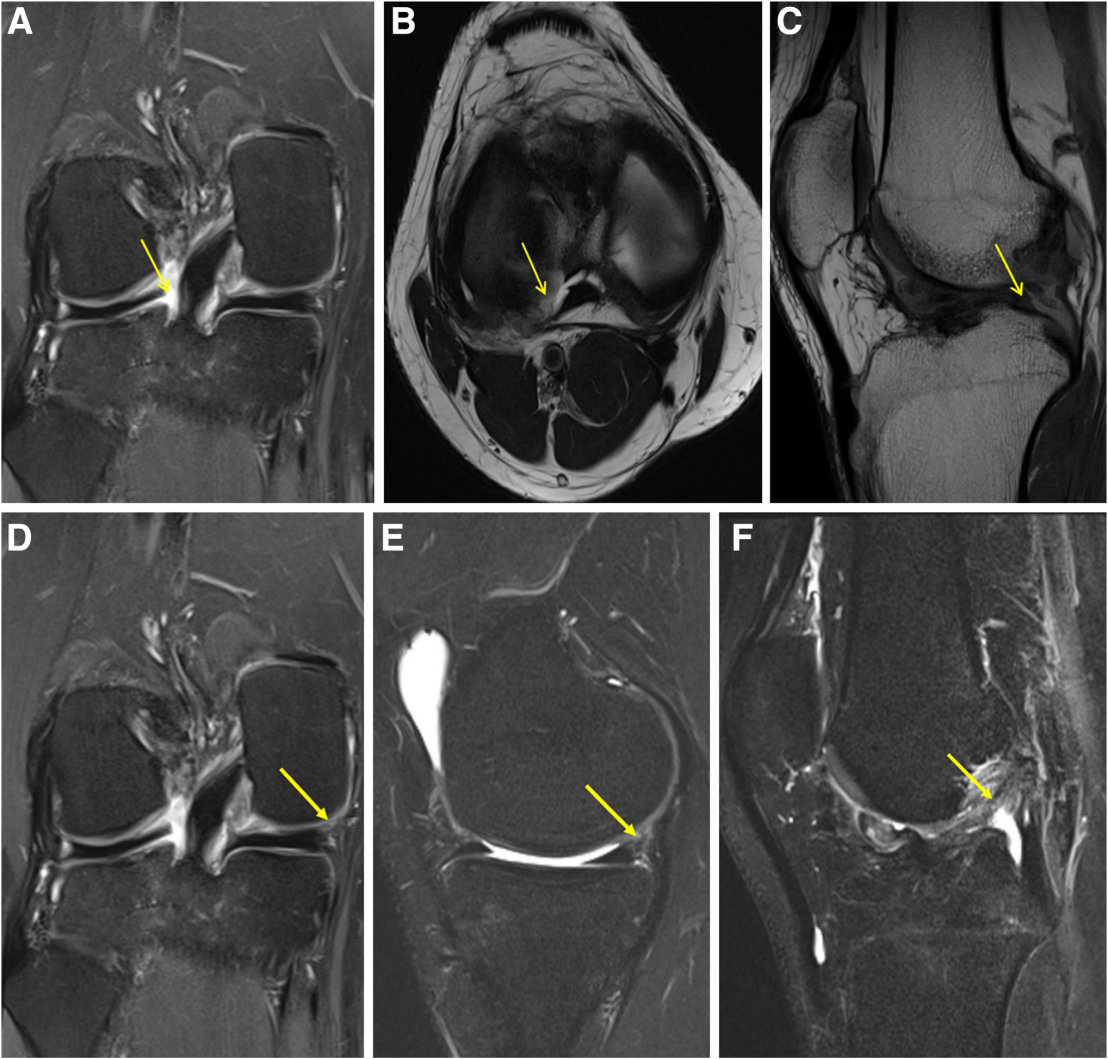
MRI of right knee in a patient with new terrible triad and ACL graft rupture. (A) Coronal T2 PD FS shows increased hyperintensity signals (yellow arrow) suggestive of lateral meniscus posterior root tear. (B) Axial T1 PD FS shows increased hyperintensity signals (yellow arrow) suggestive of lateral meniscus posterior root tear. (C) Sagittal T1 PD FS shows increased hyperintense signal (yellow arrow) suggestive of lateral meniscus root tear, also known as “ghost sign.” (D) Coronal T2 PD FS shows increased hyperintense signals in the posterior horn of medial meniscus suggestive of medial meniscus ramp lesion (yellow arrow). (E) Sagittal T2 PD FS shows increased hyperintense signals in the posterior horn of the medial meniscus suggestive of medial meniscus ramp lesion (yellow arrow). (F) T1 PD FS shows a complete tear of the ACL graft (yellow arrow). (ACL, anterior cruciate ligament; FS, fat saturation sequence; MRI, magnetic resonance imaging; PD, proton density.)
